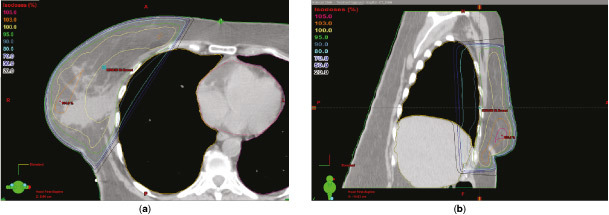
Deep inspiration breath-hold transverse (a) and sagittal (b) planning CT images from the Eclipse™ planning system (Varian Medical Systems, Palo Alto, CA). The liver (light yellow contour line) is displaced inferiorly and posteriorly away from the high 95% isodose treatment area (bright green contour line) as the right (dark yellow contour line) and left lung (magenta contour line) are fully inflated.
